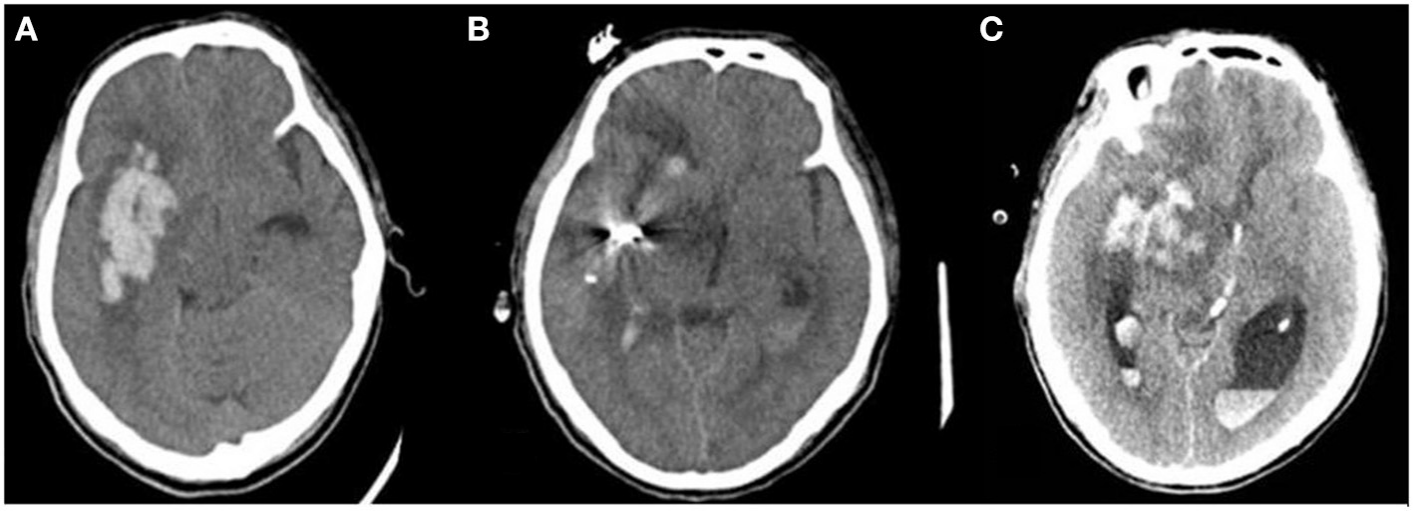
Postoperative rehemorrhage in patients with ISH following sMIS. Postoperative rehemorrhage occurred in patients with irregular-shaped hematoma. (A) hematoma before surgery; (B) Hematoma size significantly decreased on the first postoperative follow-up CT scan; (C) Hematoma size increased on the second follow-up CT scan compared to the previous CT scan, suggesting postoperative rehemorrhage.
